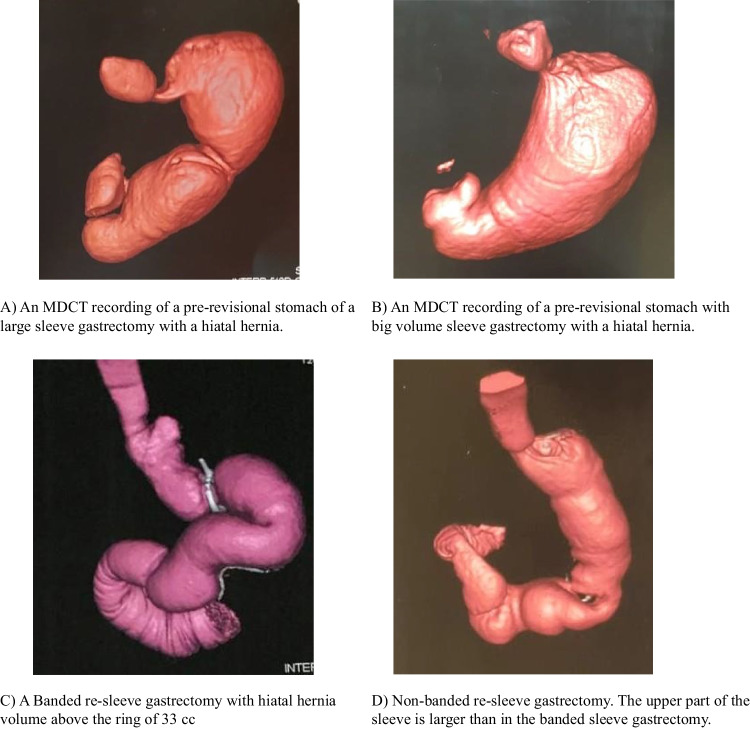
Multi-detector computed tomography (MDCT) imaging of pre- and postrevision sleeve gastrectomy
